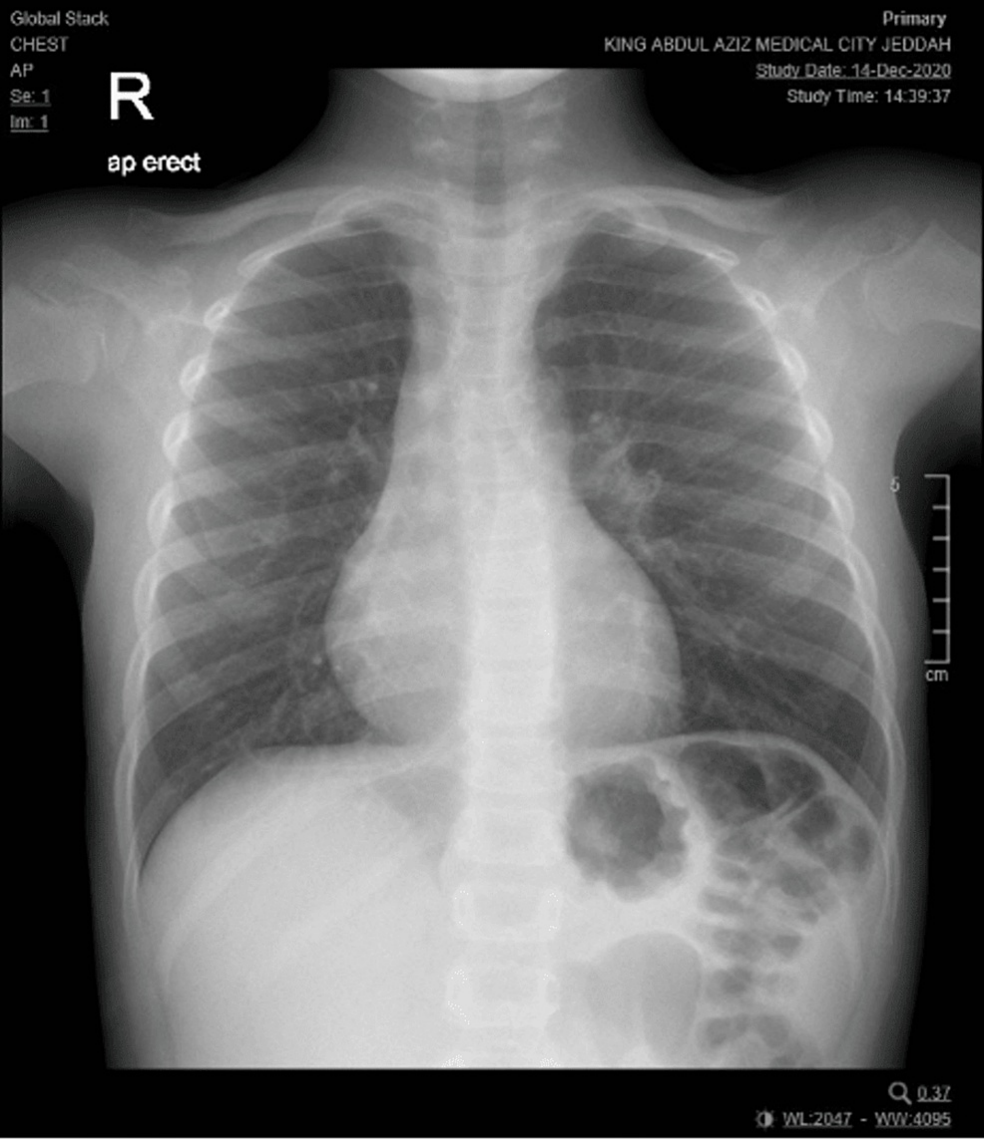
X-ray repeated at clinic
